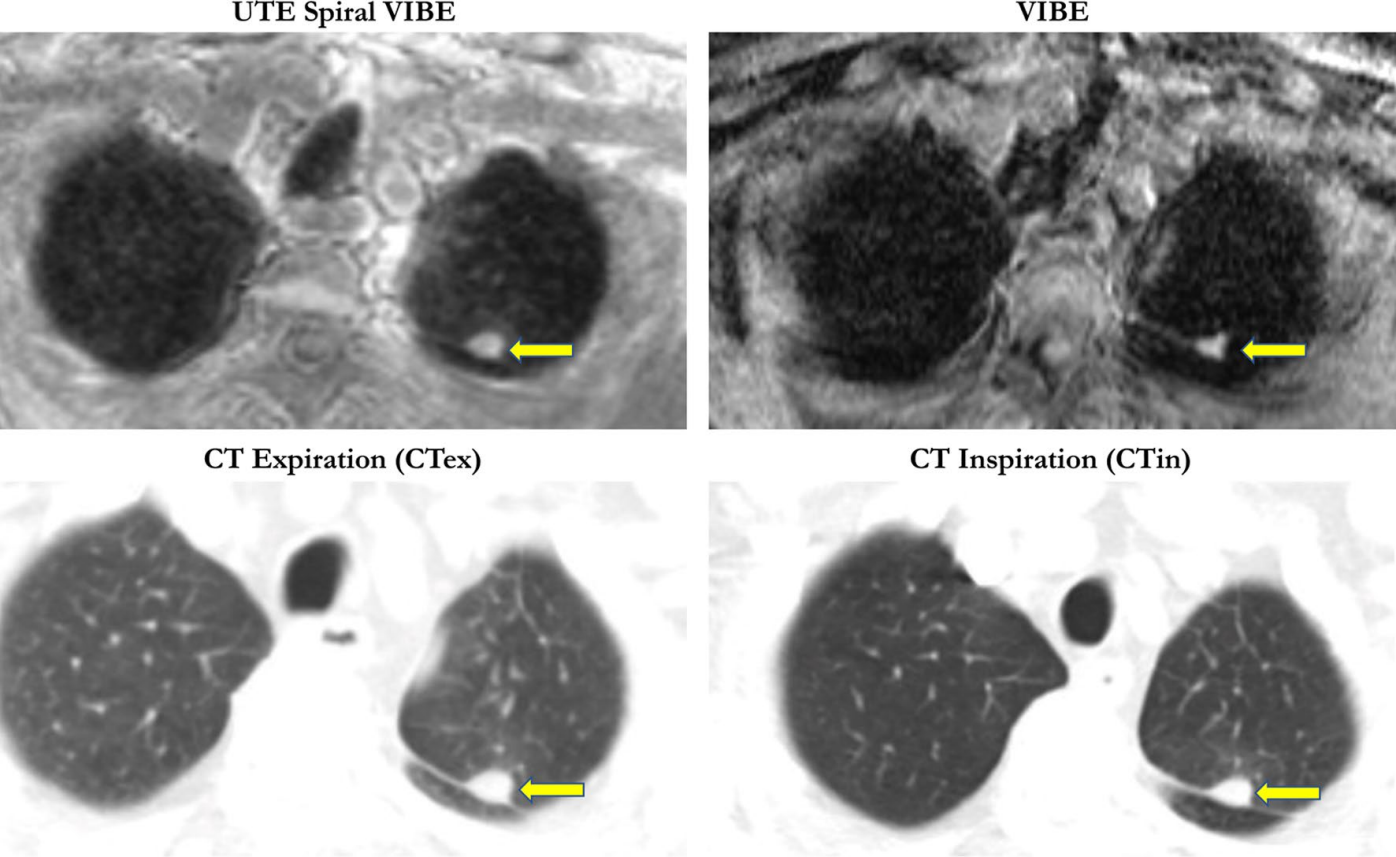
Perifissural bronchial carcinoma in the upper lobe was detected in UTE Spiral VIBE, VIBE and CT imaging; (arrow; 15 mm)
